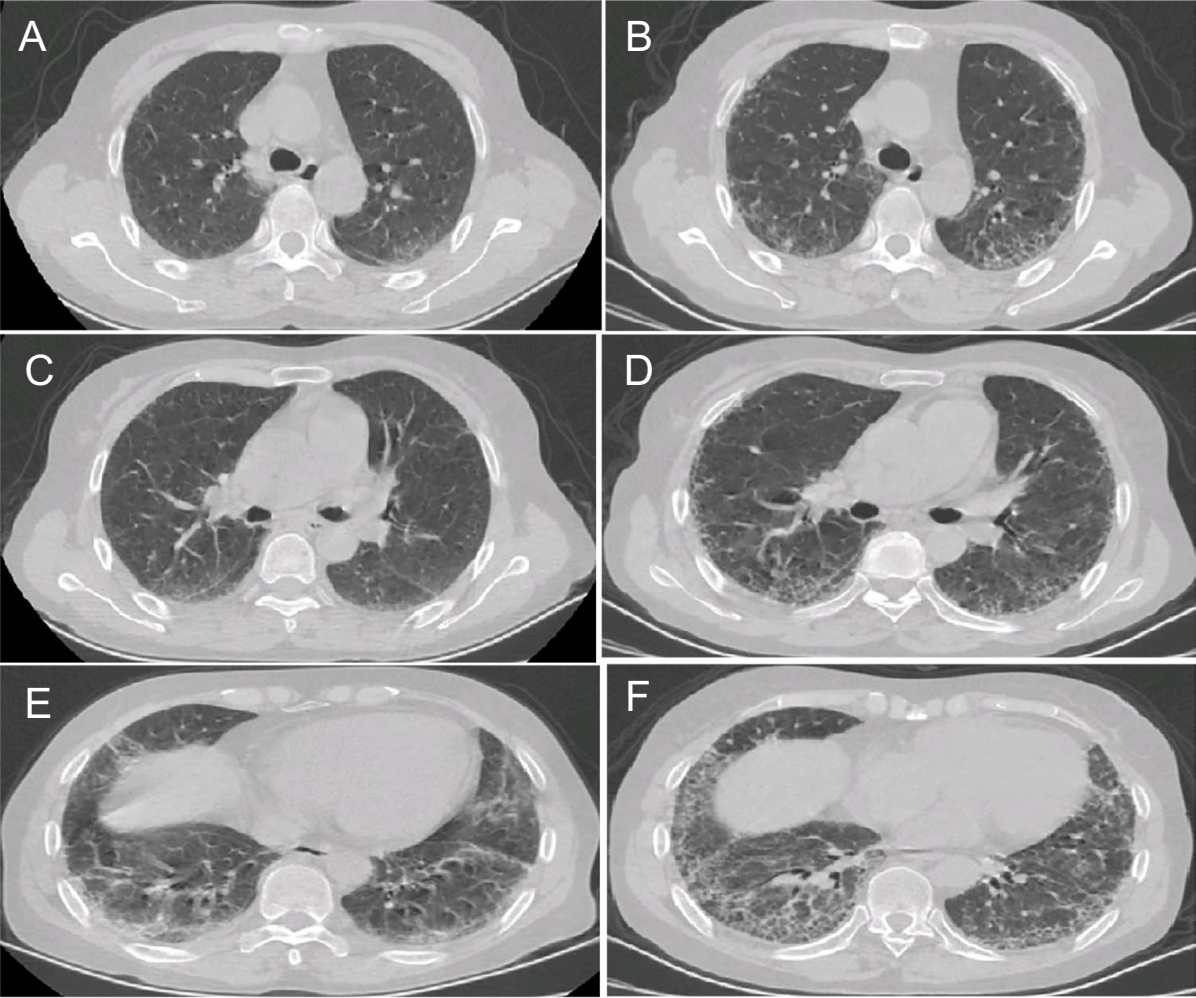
Progressive pulmonary fibrosis due to nonspecific interstitial pneumonia/organizing pneumonia overlap. A 64-year-old female with anti-PL-12 antibody-positive dermatomyositis/interstitial lung disease. A, C, E High-resolution computed tomography (HRCT) images showing consolidations superimposed on a background of ground-glass opacities in the subpleural region of the lower lung. B, D, F HRCT images showing new reticular opacities and traction bronchiectasis at the 7-month follow-up
